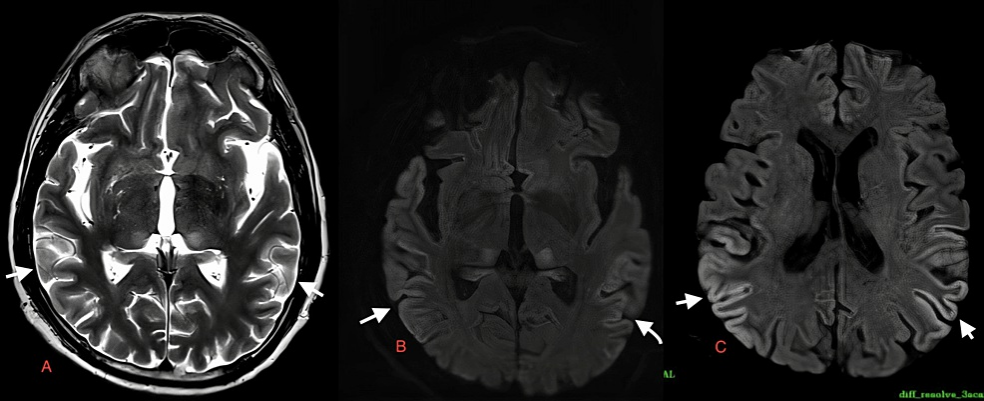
Case 6: MRI of a 15-year-old male patient on day six of admission. Increased gyral hyperintensities are observed in the posterior parietal lobe on T2W and DWI (white arrows in A, B, C). T2W: T2-weighted image; DWI: diffusion-weighted image; MRI: magnetic resonance imaging
